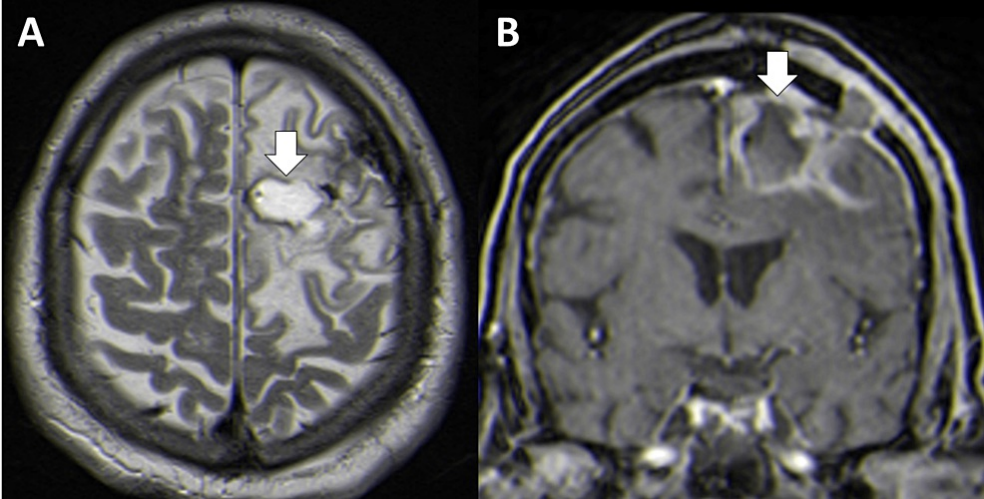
Follow-up brain magnetic resonance imaging: axial T2 (A) and coronal post-contrast T1 (B) images, three weeks after surgery, showing a residual encapsulated abscess (arrows) with perilesional vasogenic edema.
